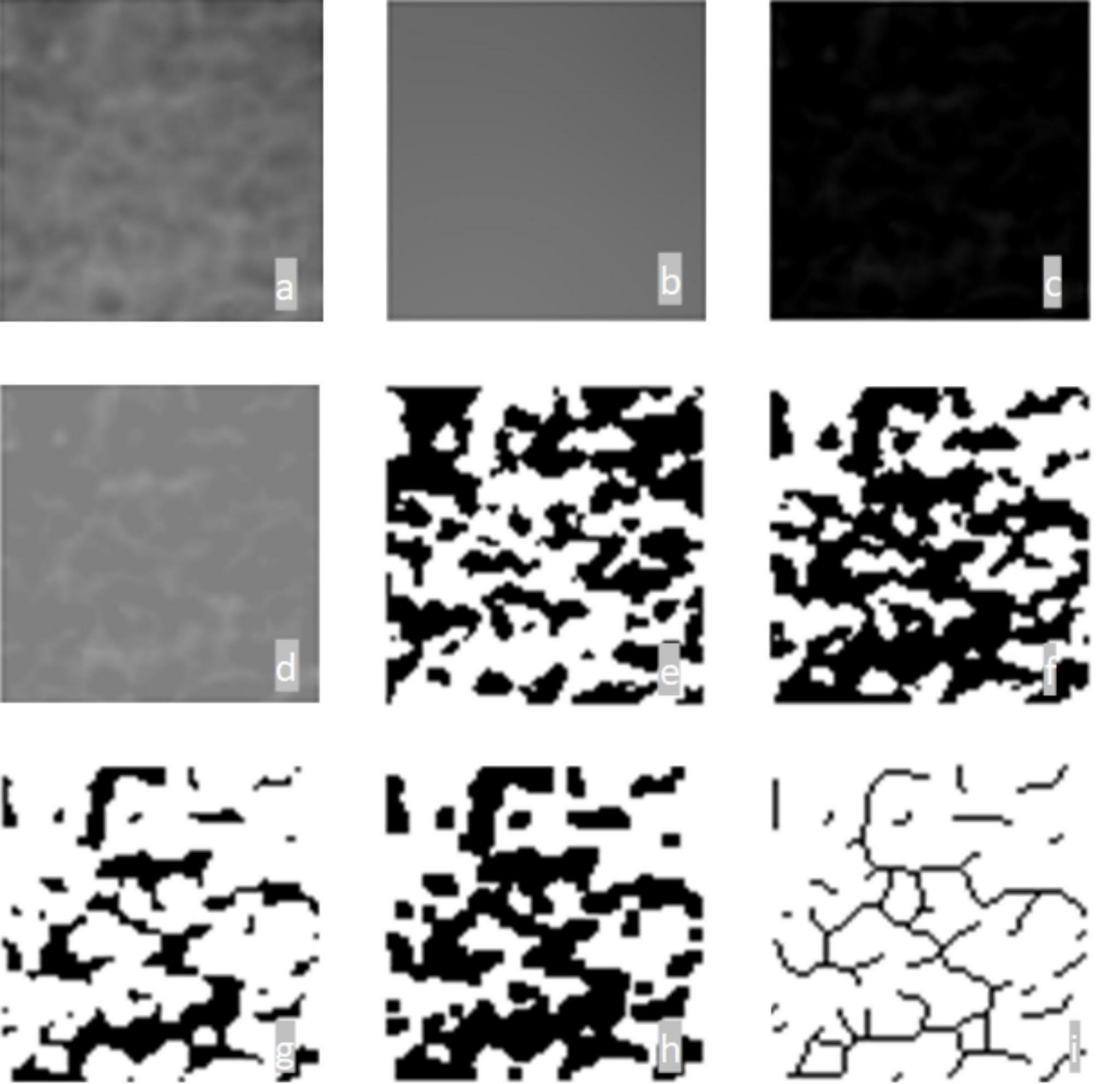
Sequential radiographical presentation of the fractal analysis with box-counting method. (A) Cropped and duplicated region of interest. (B) Gaussian blurred image. (C) Subtraction of the blurred images from the original cropped image. (D) 128 pixel added version of the image in figure C. (E) Binarized version of the image in figure D. (F) Inverted version of the image in figure E. (G) Eroded version of the image in figure D. (H) Dilatated version of the image in figure G. (I) Skeletonized version of the image in figure G
